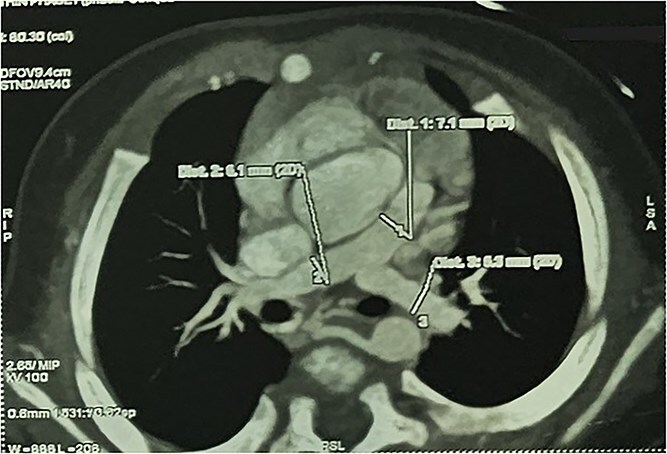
CTA demonstrating hypoplastic MPA with confluent hypoplastic branch PAs.
